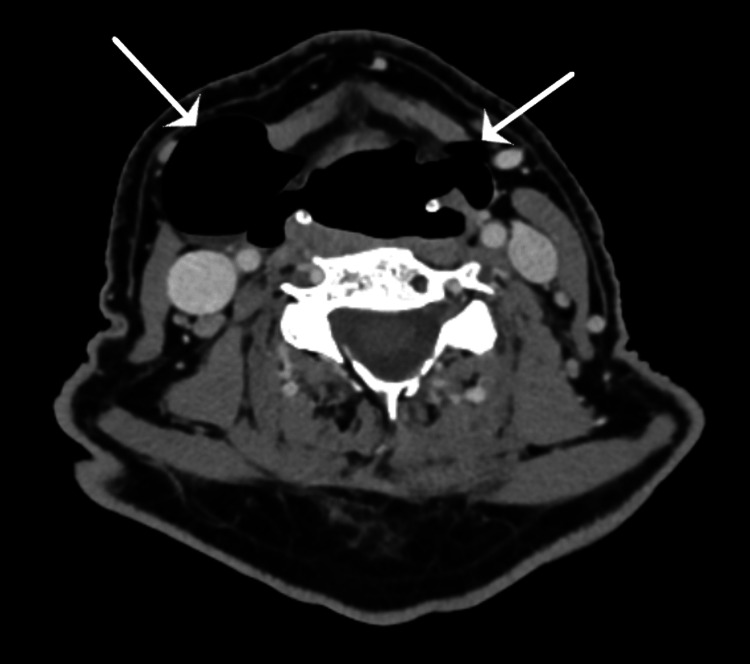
Axial slice of CT of the neck on Valsalva, which shows air-filled sacs inferior to the hyoid penetrating the thyrohyoid membrane more prominent on the right as depicted by the arrows.
